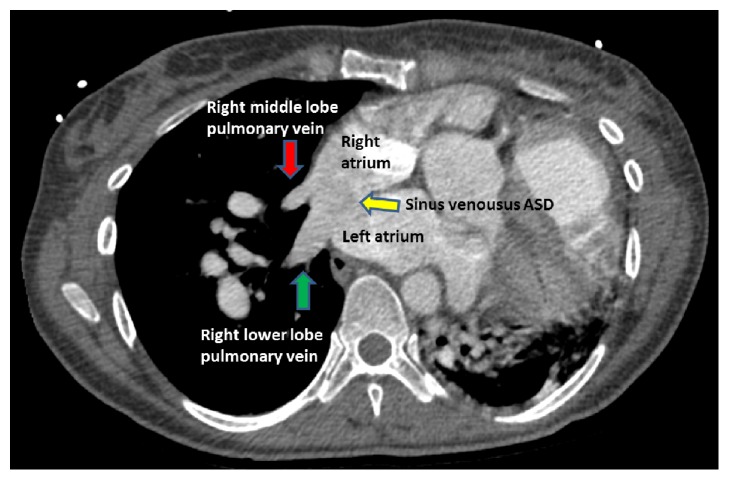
Computed tomographic angiography of the chest shows the right middle lobe vein (red arrow) draining into the right atrium (partial anomalous pulmonary venous return) and the right lower lobe vein (green arrow) entering the heart at the junction of the right and the left atria at the level of a large atrial septal defect (yellow arrow).
